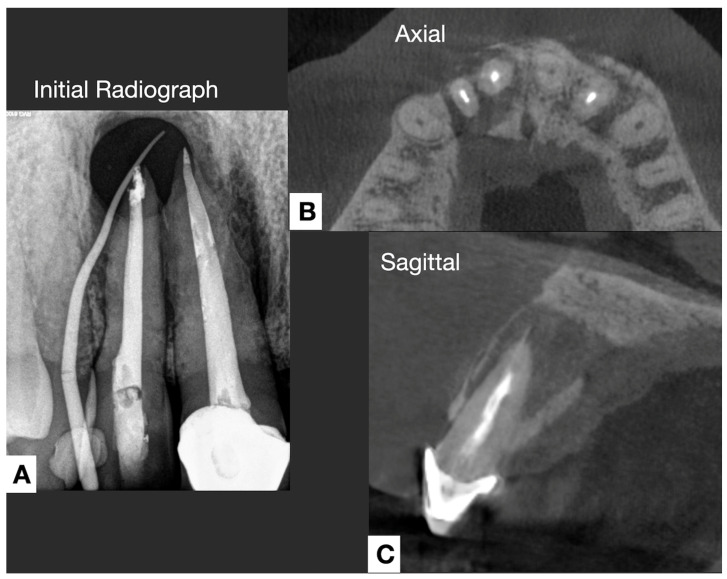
(A) Preoperative periapical radiograph of right maxillary central and lateral incisors (7 and 8) showing an extensive apical lesion encompassing the apex of both teeth and with the presence of sinus tract. (B,C) CBCT axial, and sagittal scans show a loss of bone and lingual cortical.
